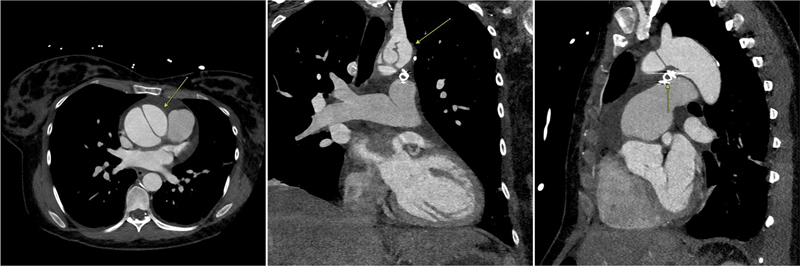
MRI images of Sibling B. Left: Axial view showing an aortic dissection flap. Middle: Coronal view demonstrating dissection extension to Zone 2. Right: Sagittal view of the ductus arteriosus with an Amplatzer Vascular Plug-2 device. MRI, magnetic resonance imaging.
